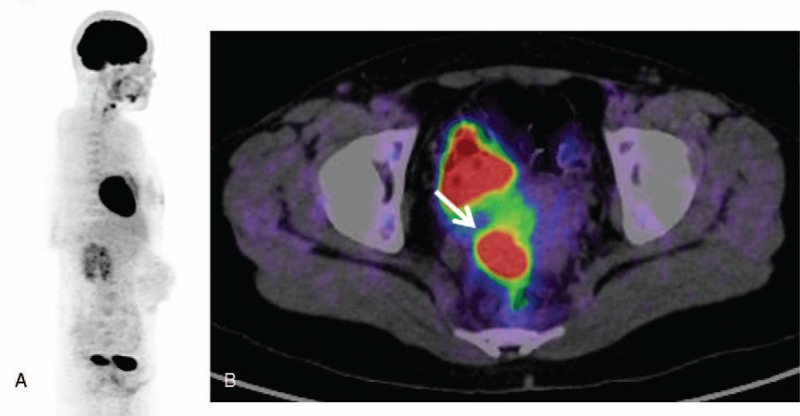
Positron emission tomography (PET)/computed tomography images of a patient with cervical cancer. (A) A typical whole-body 18F-fluorodeoxy-glucose (FDG)-PET image of a patient with cervical cancer. (B) A 32-year-old woman with stage IIA cervical cancer. FDG-PET images clearly show increased focal FDG accumulation (standardized uptake value = 22.43) in the tumor (arrow).
